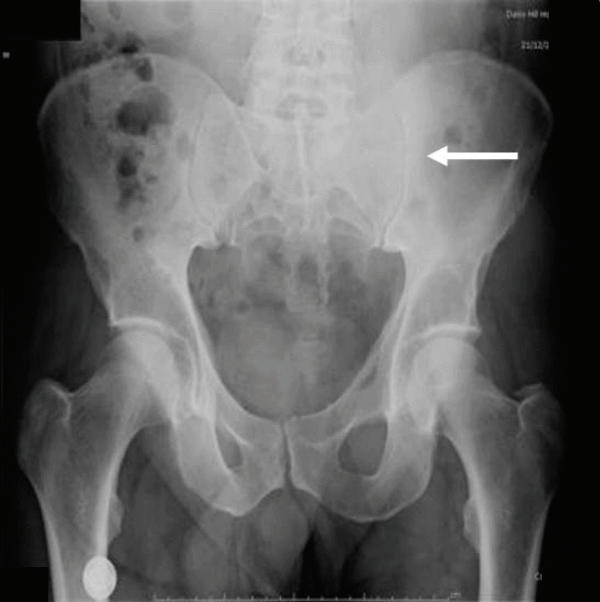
There is increased soft tissue density over the left sacro-iliac joint (white arrow). No definite bone destruction can be visualised on this plain film X-ray.
